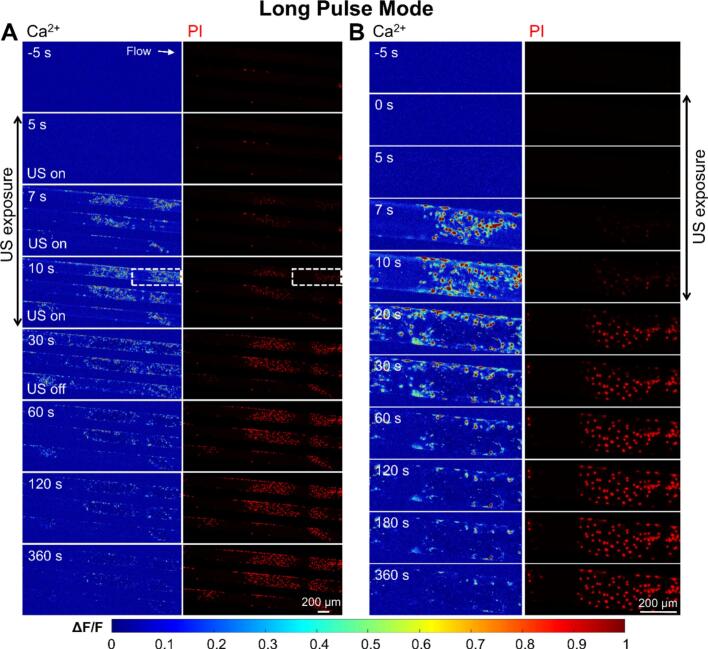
Characteristics of cellular Ca^2+^ signaling and membrane poration of bEnd.3 monolayer culture in the vessel-mimicking channel under 0.5 MPa long pulse ultrasound exposure for 10 s at a flow rate of 75 ul/min. (A) Wide-field imaging of cellular Ca^2+^ dynamics (Fluo-4, pseudo color) and PI uptake (red) in a microfluidic chip before, during (0 < *t* < 10 s), and after the long pulse ultrasound stimulation (*t* > 10  s). The left column indicates the normalized Ca^2+^ change (ΔF/F_0_) with warmer colors representing a stronger Ca^2+^ response. The right column displays PI fluorescence, highlighting membrane-compromised cells. (B) Enlarged view of the selected region from Panel A, showing the progression of cellular Ca^2+^ signaling and membrane poration at higher resolution. The same time labels and scale bars are shared between the columns in each panel.
